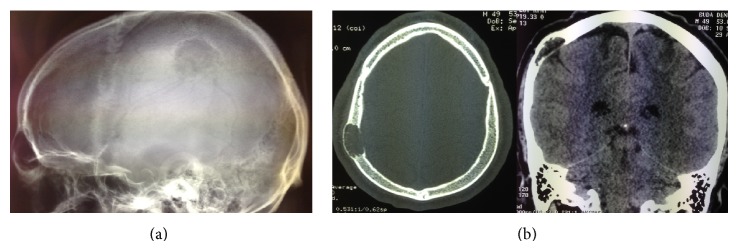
(a) Skull X-ray showing bony erosion. (b) CT brain showing inner table erosion and thinning of the outer table from the intraosseous lesion.
